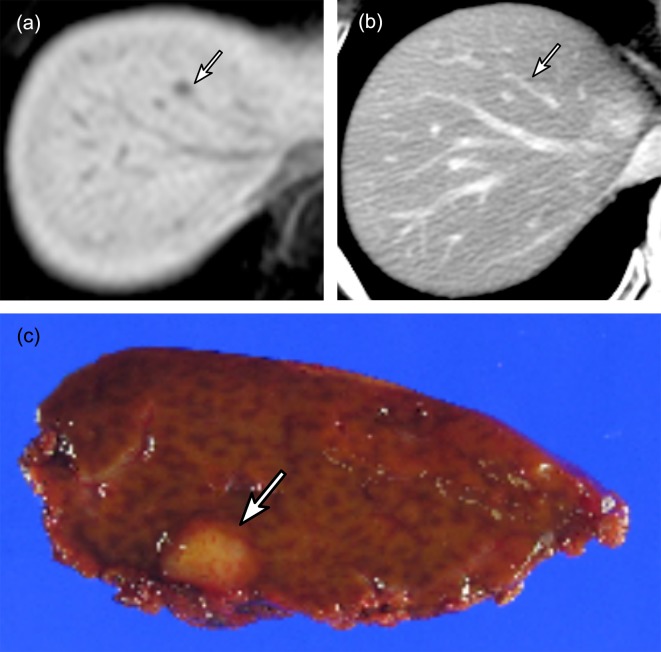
A 64-year-old woman with colorectal liver metastasis (CRLM). (a) Gadoxetic acid-enhanced transverse T1-weighted MR image during the hepatobiliary phase shows a small nodule (arrow) in segment 4. (b) The nodule (arrow) is not clearly seen at contrast-enhanced transverse CT image. (c) The nodule (arrow) measured 5 mm and was confirmed to be CRLM.
